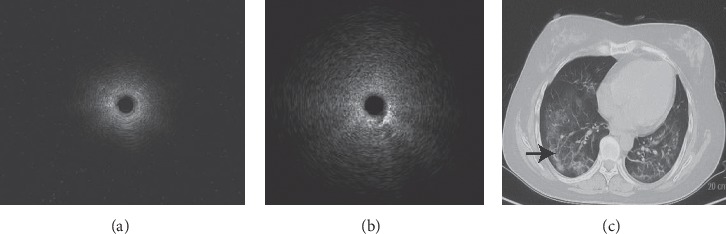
The EBUS image of normal lung parenchyma surrounding bronchial structures had patchy and numerous hyperechoic particles that were typically called snowstorm-like. The image represented normal air-filled alveoli (a), an EBUS image of Pneumocystis carinii pneumonia (PCP). The image was from a 46-year-old woman which demonstrates mixed blizzard sign, the internal echo of the lesions demonstrated diffuse heterogeneity with several hyperechoic dots and linear arcs that were distributed irregularly (b). The CT manifestations included bilateral diffuse ground-glass opacity (c). Biopsy was performed at part-solid GGO lesion in the right lower lung lobe, the diagnosis of pneumocystis carinii infection identified by mNGS ((c) black arrow).
